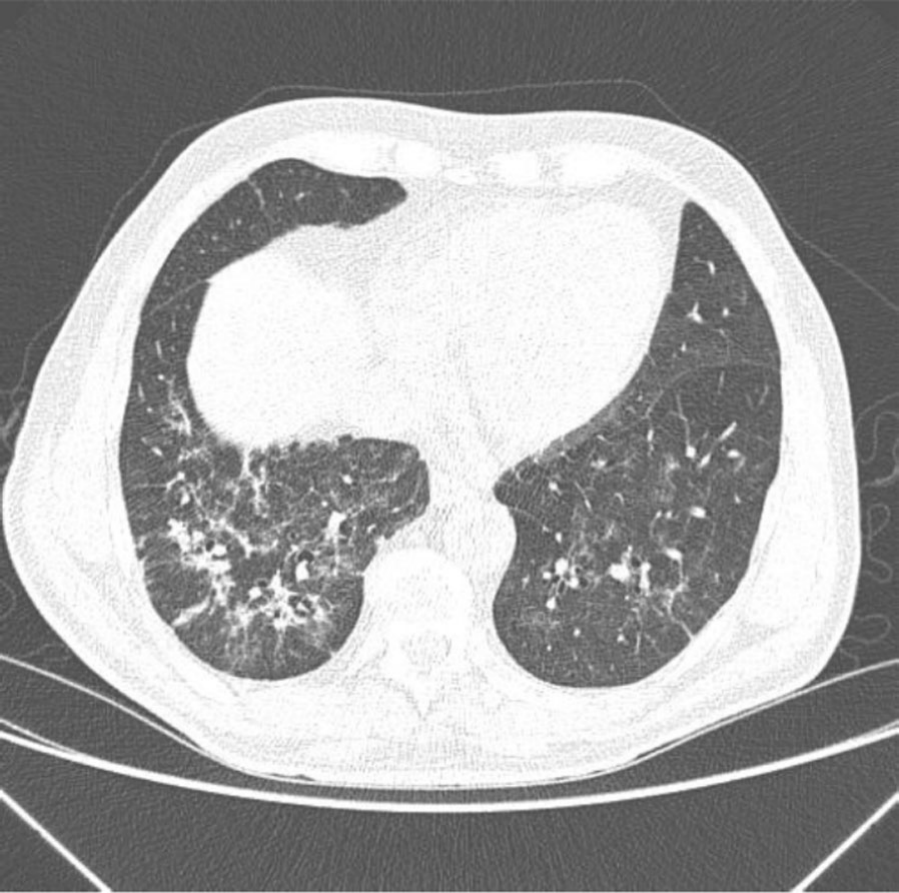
Computed Tomography of the Chest without the administration of the intravenous contrast medium—these set of findings is suggestive of the inflammatory process, and the viral etiology must be included in the etiological differential, particularly COVID-19. Regarding the PET-CT 29/04/2020 exam, there was an increase in the extension and attenuation of pulmonary opacities
